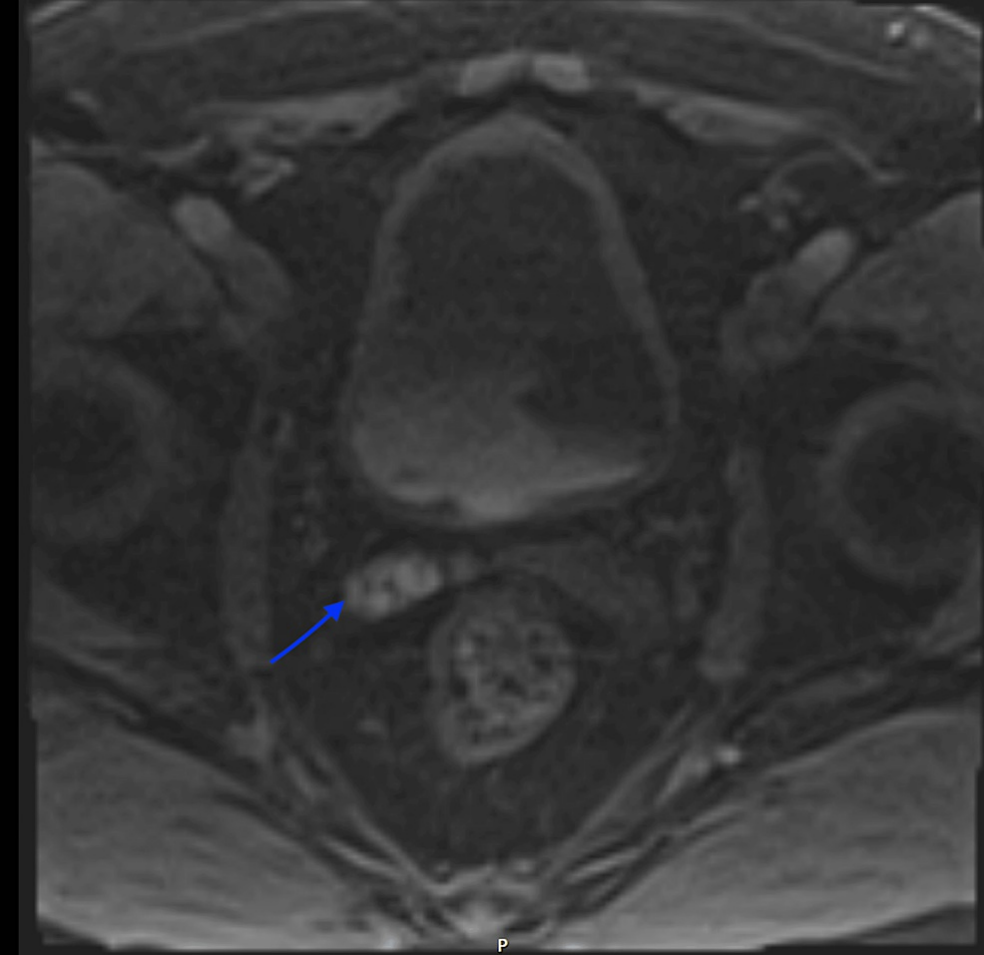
T1-weighted MRI showing high signal intensity in the right seminal vesicle (arrow)
